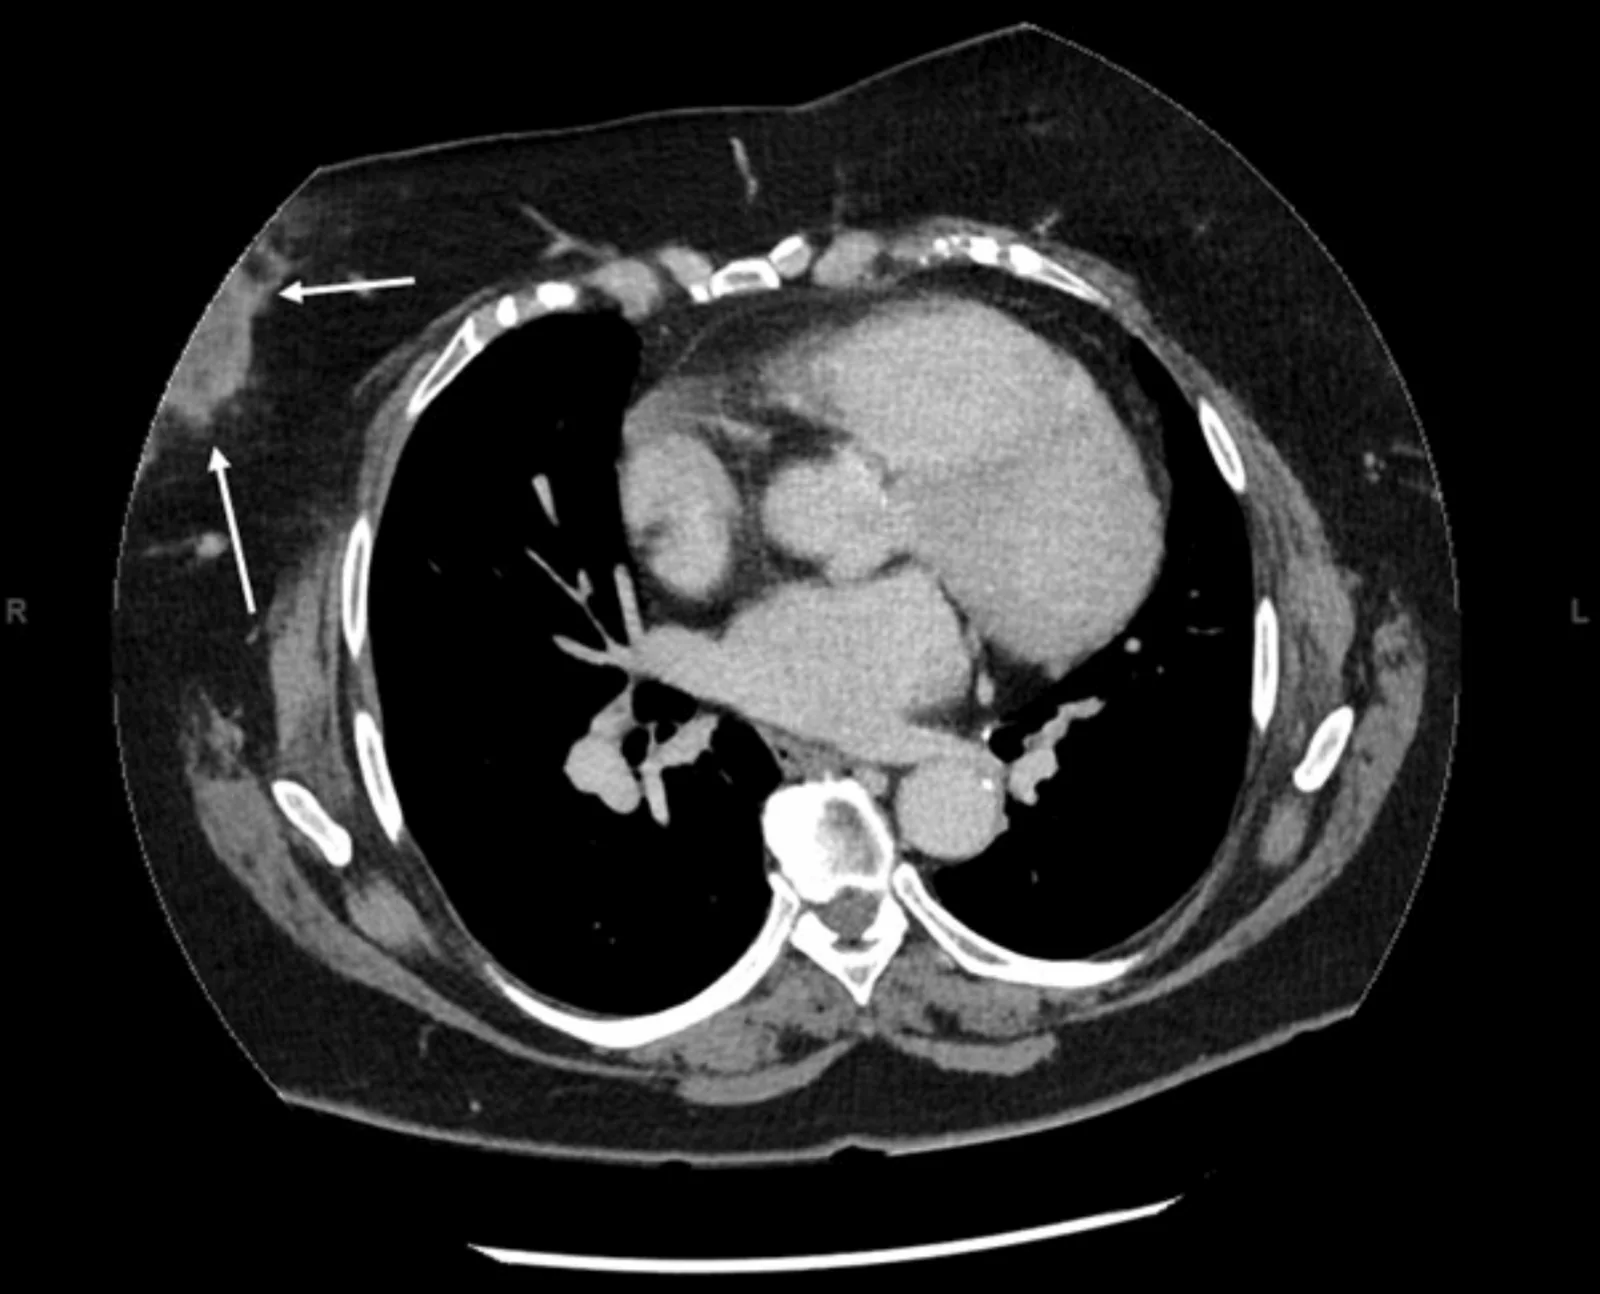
Follow-up axial chest CT performed six months later showed persistent abnormalities in the right breast (arrows), with resolution of the pulmonary findings.
